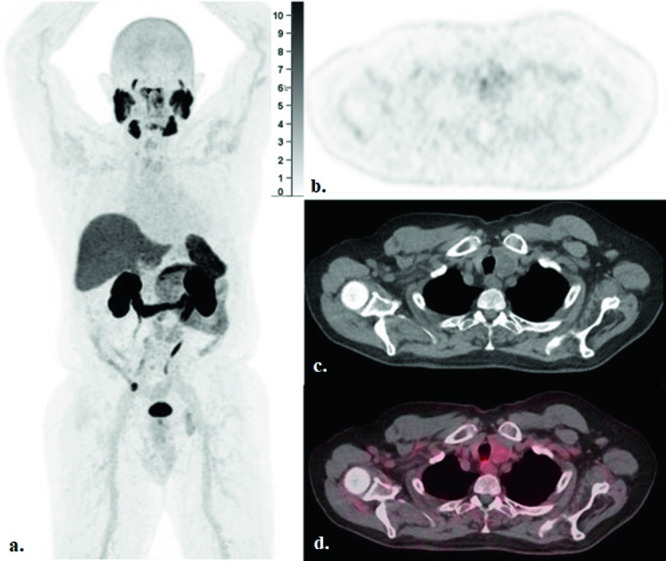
A prior F-18 DCFPyL PET/CT imaging obtained about 20 months since progression on rucaparib while patient was on ADT monotherapy, with concurrent prostate-specific antigen level of 25.4 ng/ml after systemic and radiation therapy in winter 2022. a Maximum INTENSITY PROJection (MIP), b Axial PET, c Axial CT and d Axial fused PET/CT images showed hypodense partially calcified 3.1 × 2.2 cm left thyroid nodule without notable radiotracer uptake (SUVmax: 3.6) (arrow).
